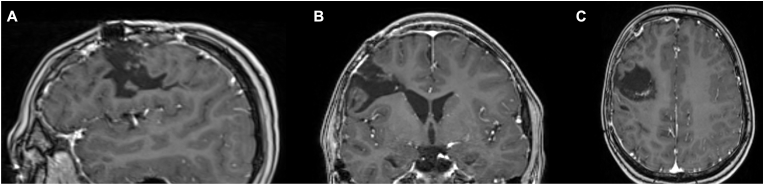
Follow-up MRI. The follow-up MRI showing no remnants of the arteriovenous malformation is depicted in sagittal (A), coronal (B), and axial (C) views.
